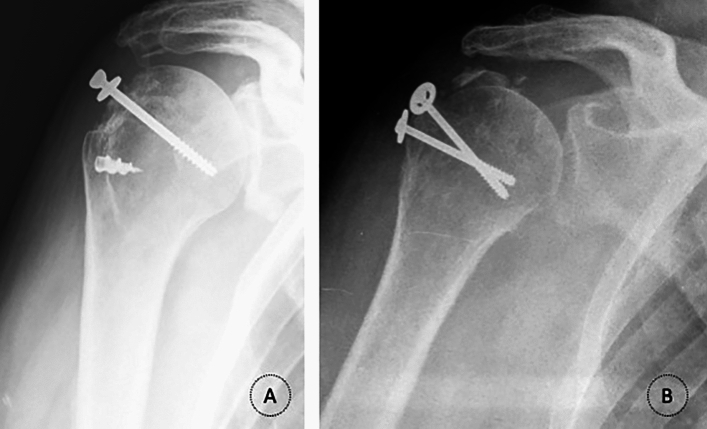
Radiographs displaying examples of complications. A Screw pull-out; B interfragmentary screw failure with greater tuberosity ascension
